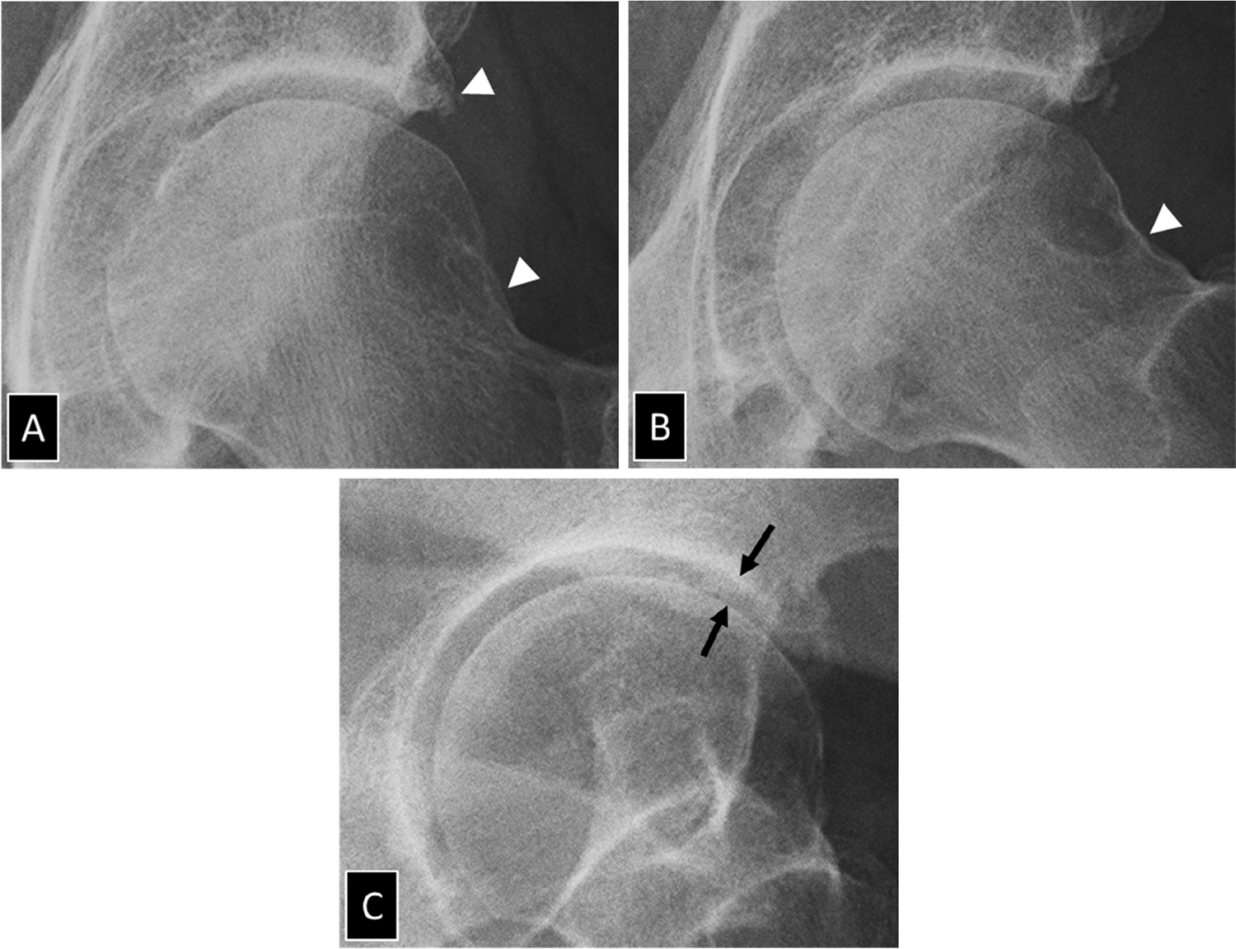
Value of Lequesne’s false profile view in a 65-year-old man with mild hip pain after walking. A AP and B Lauenstein lateral radiographs demonstrate almost normal joint space and osteophytes (arrowheads). C Lequesne’s false profile demonstrates almost 50% joint space narrowing in the anterior aspect of the joint (arrows) that cannot be seen on the other radiographs
